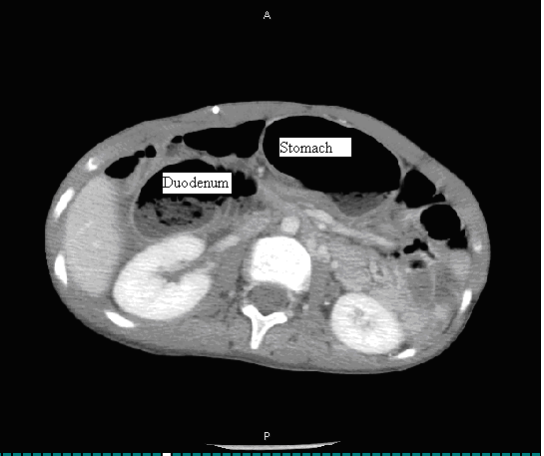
Abdominal computed tomography scan shows dilated stomach, duodenum and duodenal stenosis.
